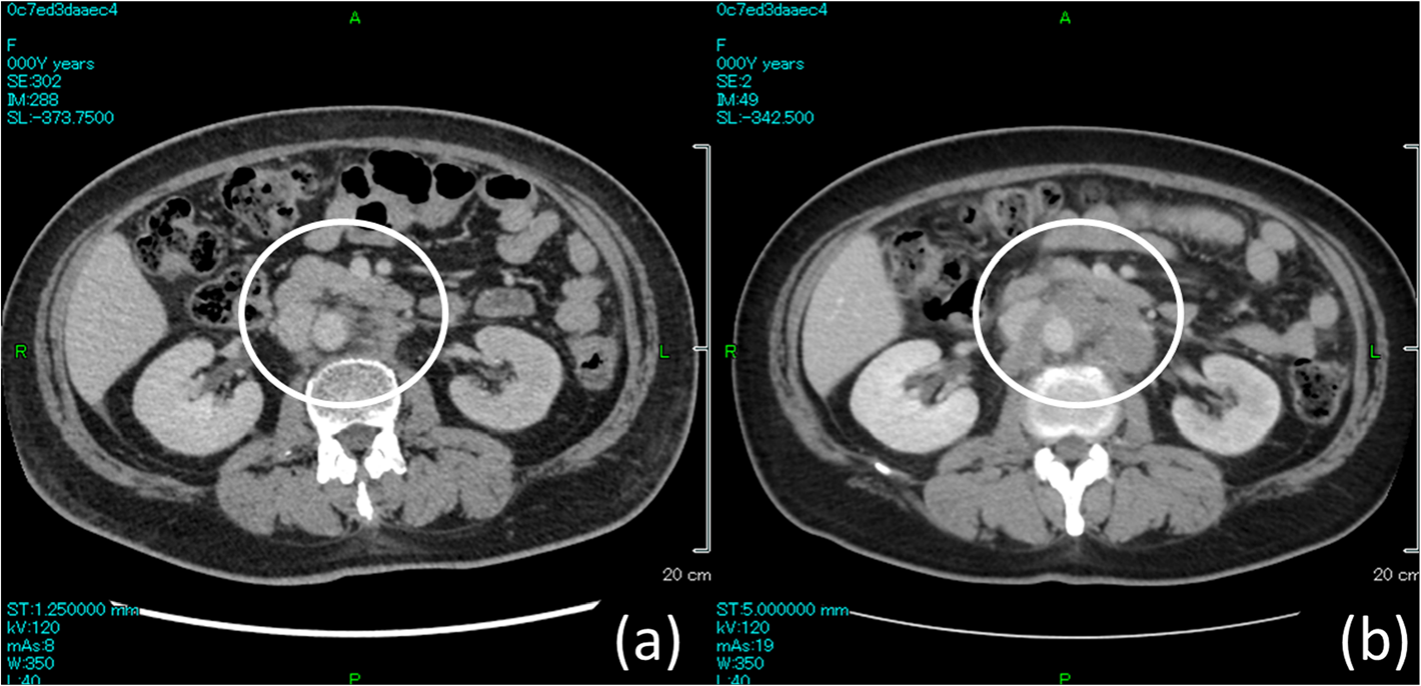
Abdominal computed tomography (CT) images of Case 4. The metastatic para-aortic lymph nodes (in circles) had shrunk after three cycles of chemotherapy (a), but re-growth occurred during the delay in treatment (b)
